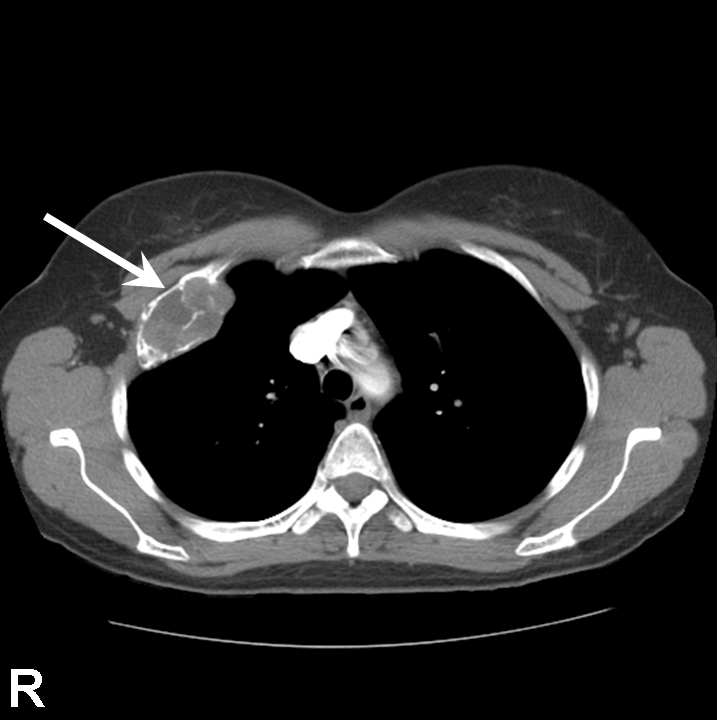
Computed tomography scan of chest (case no. 2) demonstrating a radiolucent lesion of the right anterior second rib with cortical bubbling or blebbing, and without radiographic evidence of an acute fracture, calcification or malignancy (arrow). R indicates right side.
